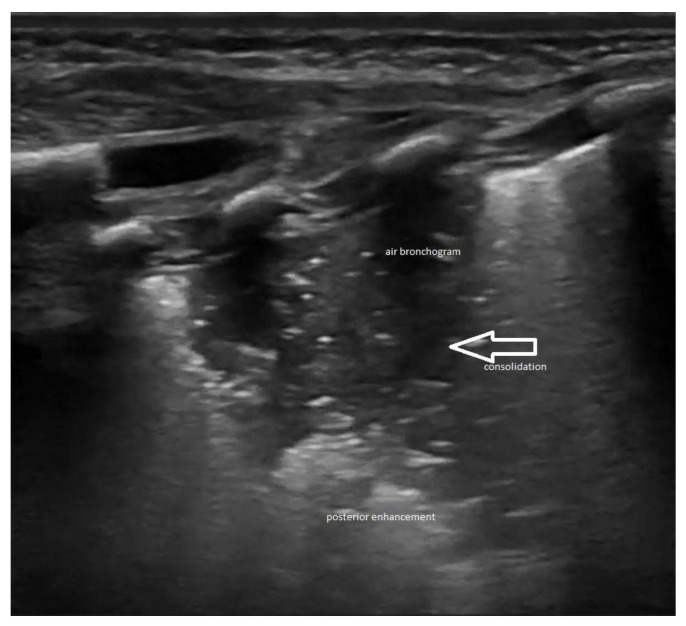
Lung ultrasound appearance in congenital pneumonia. Consolidation—arrow, air bronchogram—white spots and posterior enhancement with anfractuous appearance—shred sign.
